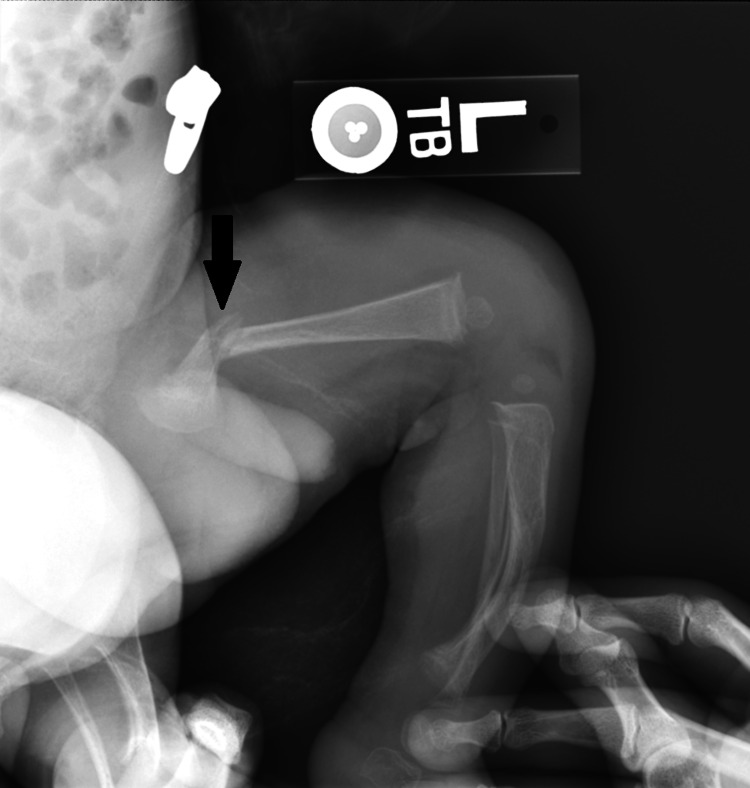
Radiographic view of the patient’s index fracture of the left femur, obtained after an unknown traumatic event to the left lower extremity. The black arrow denotes the fracture site.
